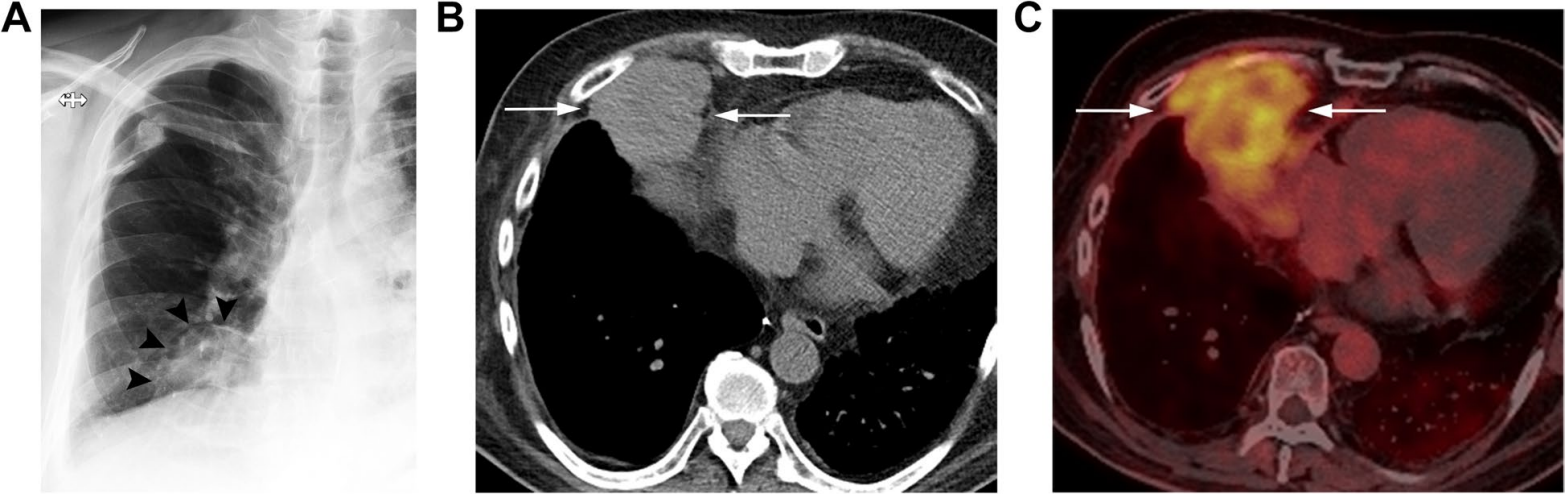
PTLD in a 72-year-old male 1 year out from single lung transplant. A Frontal radiograph shows a mass in the medial right lower lung (arrowheads). B Axial unenhanced CT (mediastinum window) shows a circumscribed soft tissue density mass (arrows) in the right cardiophrenic angle. C Axial PET-CT shows heterogeneously increased FDG uptake in the mass (arrows). No other FDG-avid lesions were identified. Tissue sampling showed monomorphic PTLD, which was EBV positive
